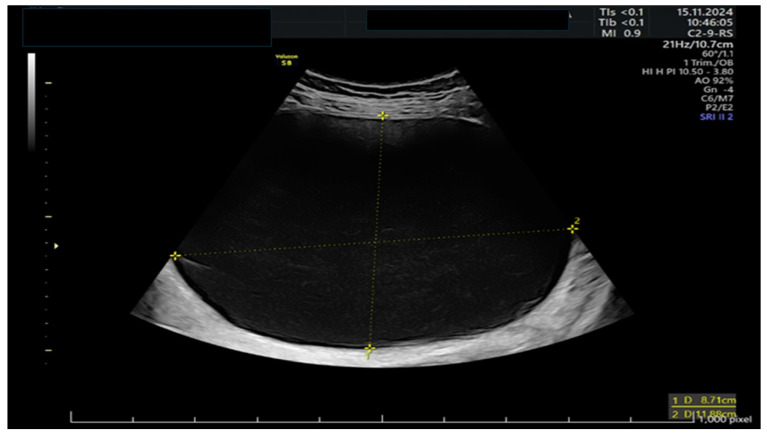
Detail of the major cystic compartment on ultrasound.
